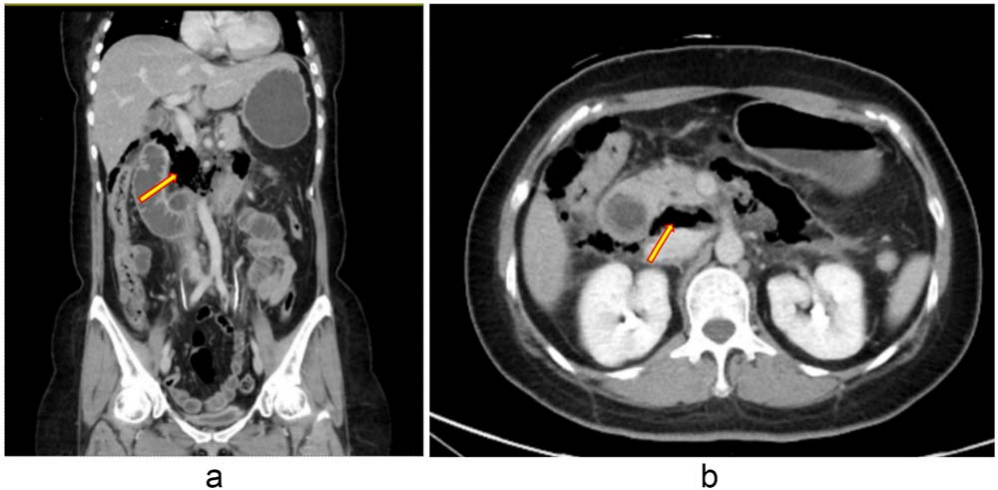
(a, b) Contrast-enhanced abdominal CT scan (26 August 2025). Imaging demonstrates retroperitoneal free air with suspicion of perforation in the retroperitoneal region.
